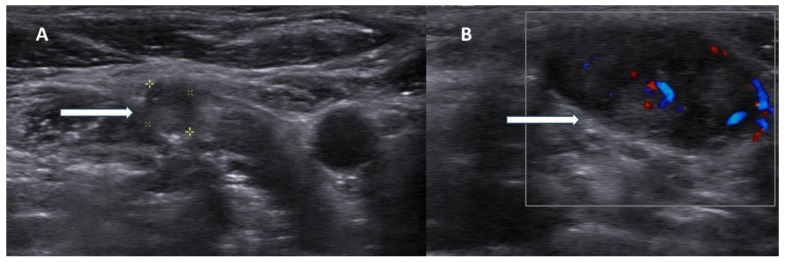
Ultrasonography images of parathyroid carcinoma neck metastases. Legend: 70-year-old patient presented with parathyroid carcinoma lymph node metastases with hypoechoic lesions without fatty hilum ((A), white arrow) and chaotic blood flow in Doppler imaging ((B), white arrow).
